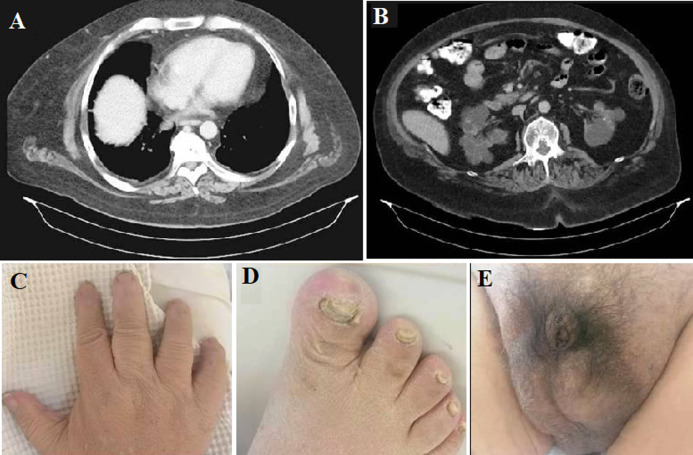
computed tomography findings and clinical characteristics of a 52-year-old patient with Bardet-Biedl syndrome; A) mediastinal lipomatosis; B) renal hypoplasia; C) brachydactyly of fingers; D) brachydactyly of toes; E) hypogonadism (micropenis)
